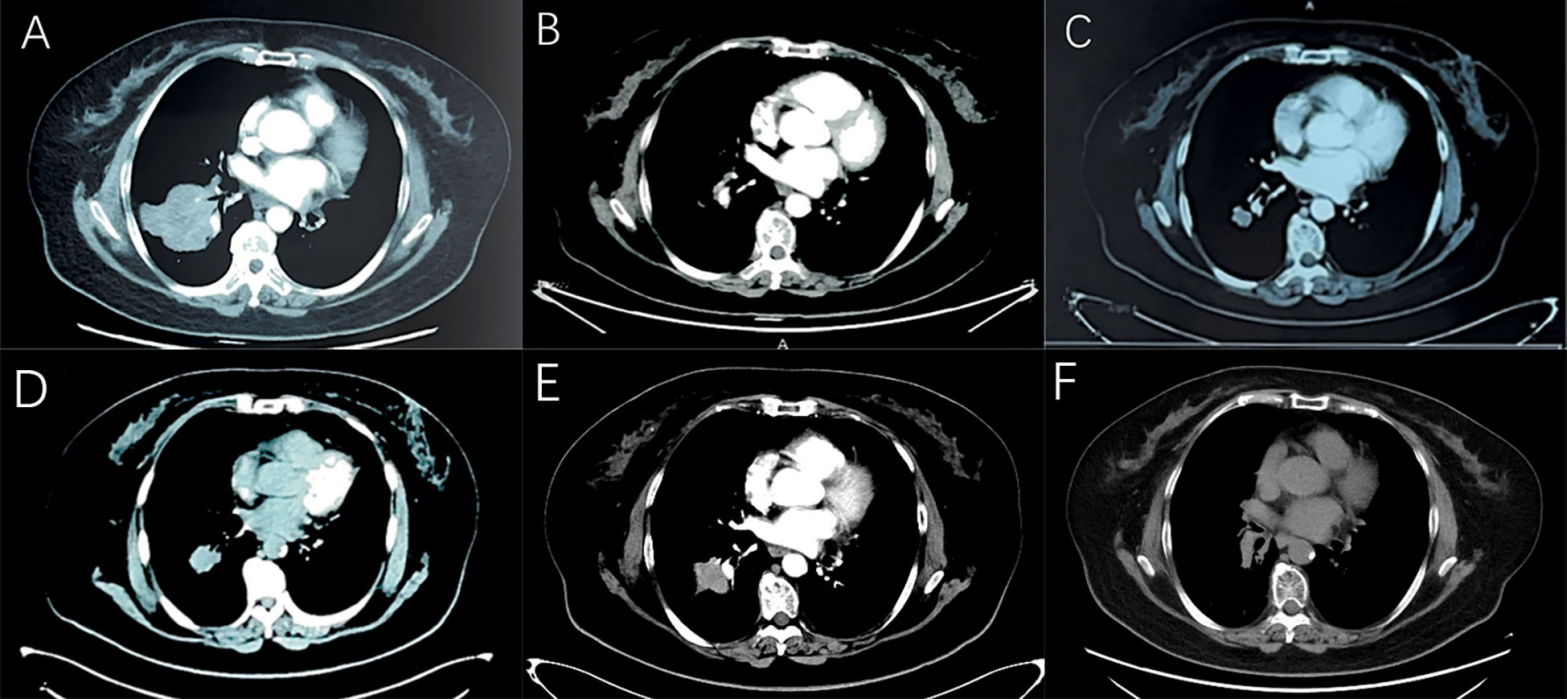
Computed tomography. (A) There is an obvious occupying lesion in the right lower lobe. (B) Targeted treatment with osimertinib resulted in a cCR. (C) Two years later, a space-occupying lesion reappeared, and the treatment efficacy was evaluated as PD. (D) Targeted treatment with furmonertinib resulted in disease progression. (E) Treatment with sindilizumab combined with bevacizumab and PC chemotherapy regimen resulted in disease progression. (F) Treatment with duvatolimab combined with EP chemotherapy regimen and local radiotherapy resulted in a PR.
